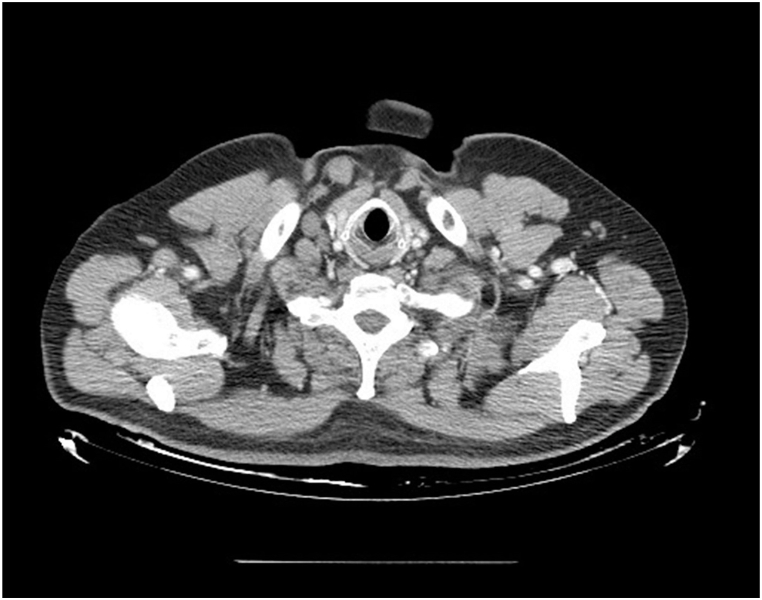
A CT scan of the neck revealed metastasis in the right lymph node of the neck level IV and the right lymph node of the neck level VB.
